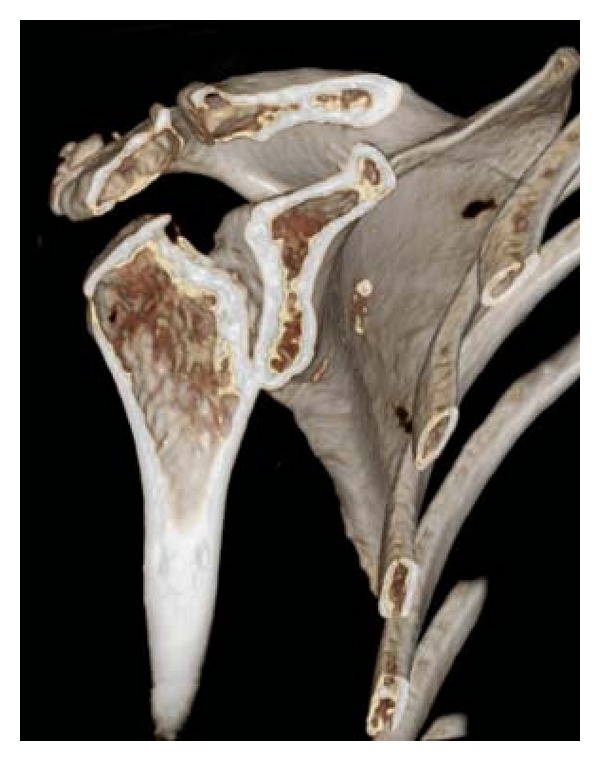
3D reconstructed CT scan showing osteonecrosis of the humeral head, the acromion, lateral clavicle, and the glenoid in Gorham-Stout disease.
